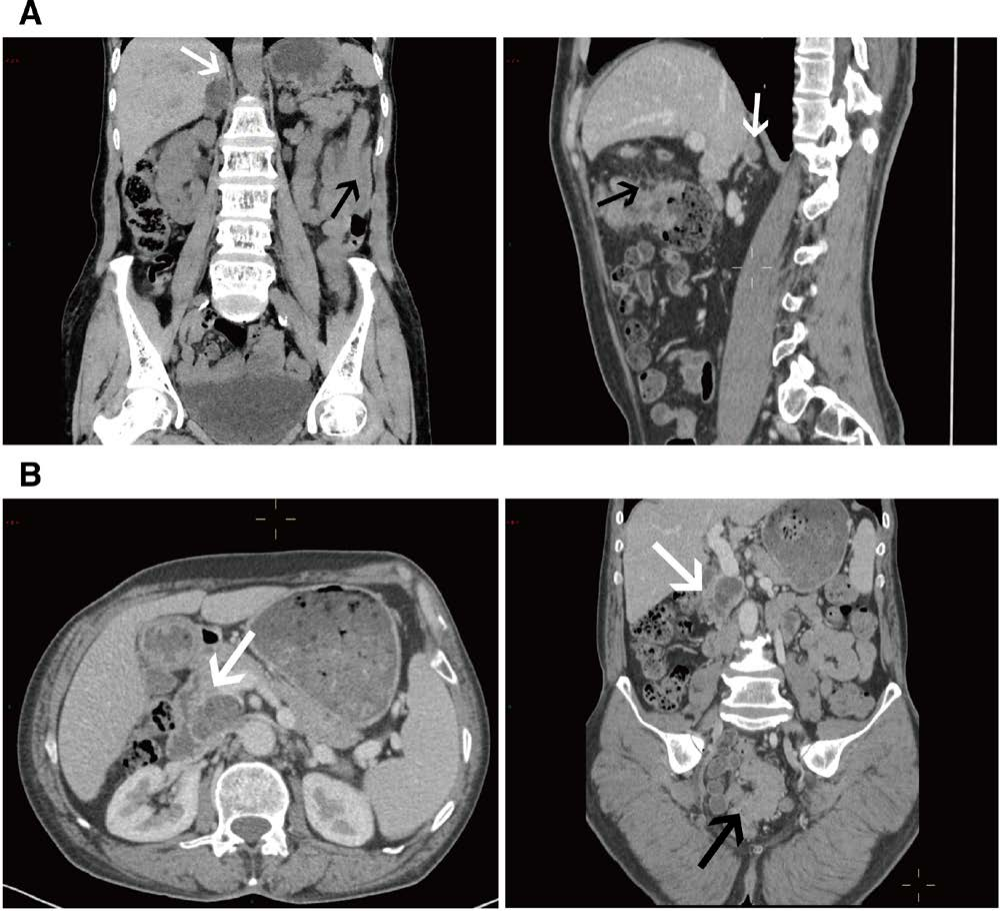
(A) Adrenal incidentalomas. Two patients with colon cancer (black arrows) and adrenal nodule (white arrows) in the right adrenal gland. (B) Pancreas incidentalomas. A 61-year-old man with 5.5 cm rectal cancer (black arrow) and a 2.2 × 3.8 cm irregular low-density mass (white arrows) of pancreas on transverse and coronal CT images.
